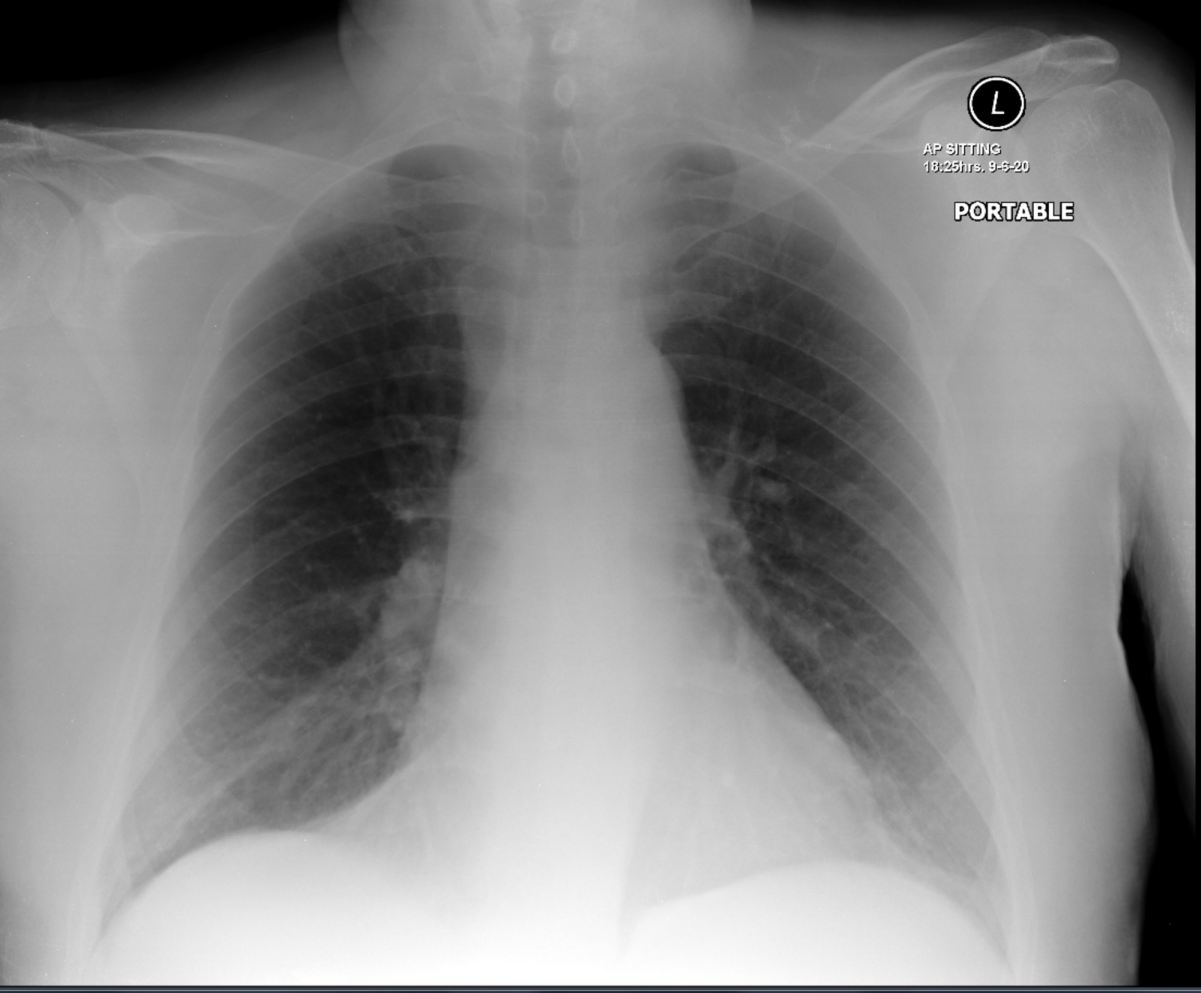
Patient’s chest X-ray showing normal appearance.
